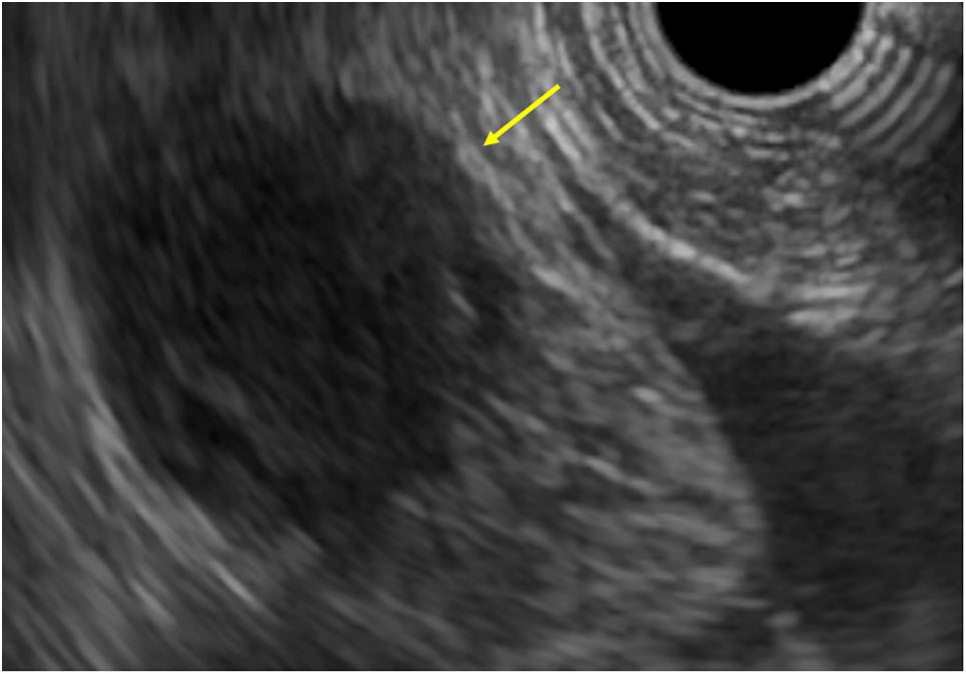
Endoscopic ultrasonography showed a hypoechoic mass with a slightly hyperechoic central component, which were difficult to distinguish (arrow)
